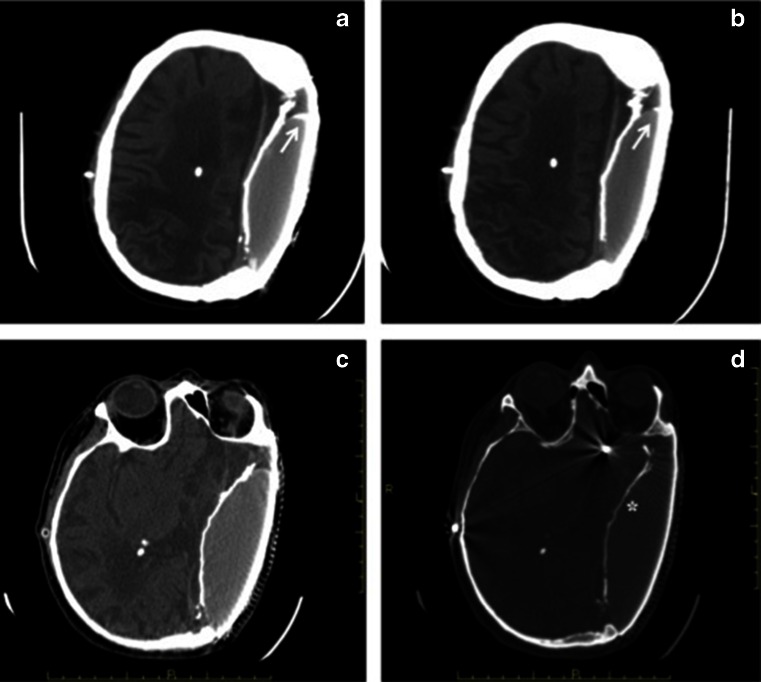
Axial CT with different window levels: soft tissue (a–c) and bone window (d). CT scan shows a white, high attenuation ring, indicating that implant shell is intact. The silicone density is homogeneous and there are no CT signs of rupture, neither wrinkling nor infolding of the implant shell (a–c). There is also a focal calcification of the capsule on the anterior aspect of the shell (*white arrow*). Both the anterior and posterior margins are smooth with rounded shaped angles with the metallic mesh (c). Medially to the implant there is a calcified Lyodura (*asterisk*). Right V-P shunt is seen. Enlargement of the supratentorial ventricular system and prominent subarachnoid space surrounding the right cerebral hemisphere
